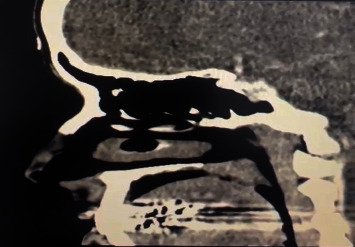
Computed tomography demonstrating isolated poorly defined soft tissue swelling within the right-sided lateral nasal wall anteriorly. There was a mild heterogeneous contrast enhancement but no clear expansion to surrounding structures.
